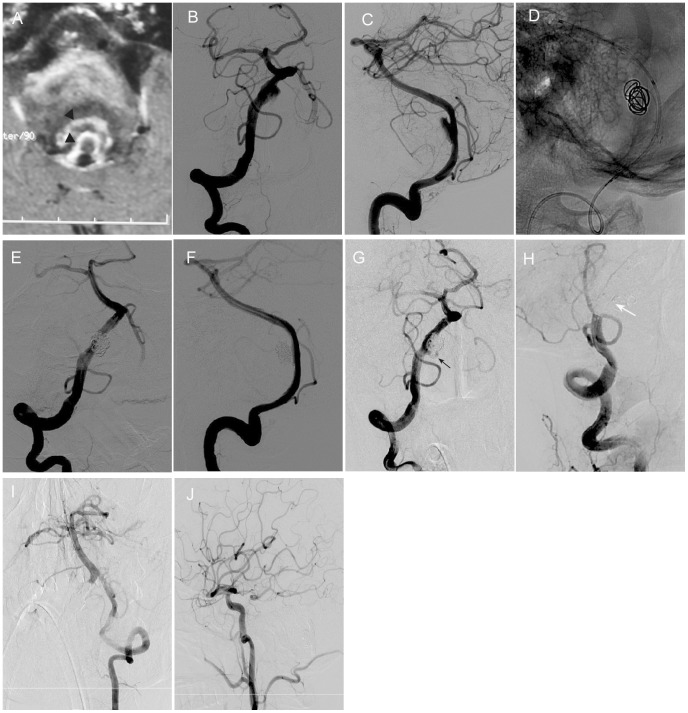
Retreatment with endovascular occlusion with aneurysm trapping was performed for recurrent huge intracranial VADA after multiple stents assisted coiling. A 54-year old male presented with headache and dysphagia. MR imaging showed huge onion skin-like thrombus (black arrowhead), intramural hematoma and compression to brain stem (A). Right vertebral angiograms of frontal (B) and lateral (C) view showed a dissecting aneurysm. Multiple stents assisted coiling (D) were performed with complete occlusion (E, F). Follow up angiography (G) after three months revealed regrowth of original dissecting aneurysm (black arrow). Retreatment by endovascular occlusion with aneurysm trapping (white arrow) (H) was performed. Collateral blood flow could be seen from contralateral VA (I) and left posterior communicating artery (J).
